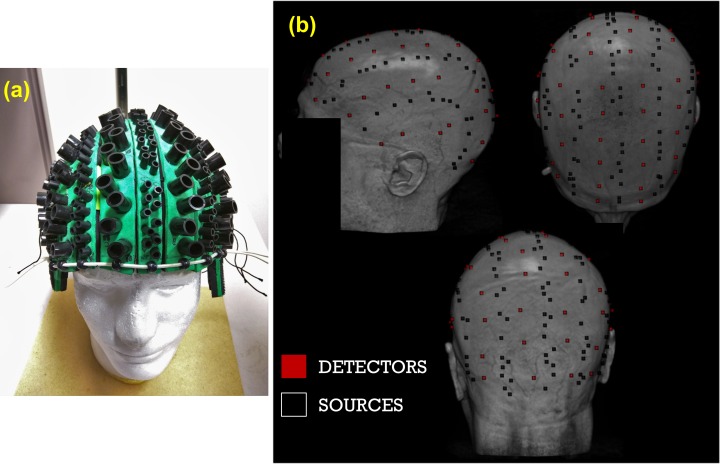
(a) Photograph of the soft foam, adjustable optical recording cap used in the study. Detectors were fitted into the larger tubes while sources were fitted into the smaller tubes. The cap was further secured to the subject’s head by tightening an elastic band around its circumference of the cap and through the usage of a custom-built chin strap. (b) Locations of sources (black) and detectors (red) digitized in one representative participant, and plotted over the corresponding surface-rendered structural MRI (left, top and back views).
